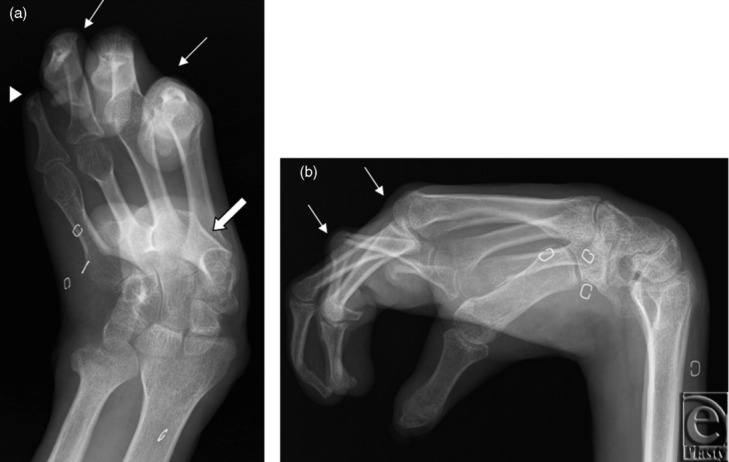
Right hand radiographs. Surgical planning posteroanterior and lateral (*a*, *b*) radiographs of the right hand of subject 1 demonstrate flexion deformity of the wrist and amputation of fifth finger at the proximal interphalangeal (PIP) joint (arrowhead). Flexion deformities of the second and third metacarpophalangeal joints and second through fourth PIP joints are present (arrows). The thumb is held in adduction (block arrow).
